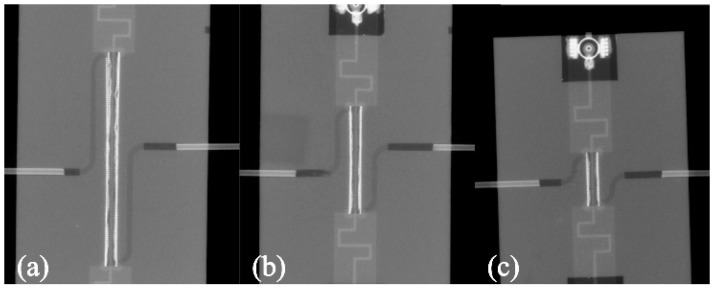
X-ray images of (a) 20 mm, (b) 10 mm, and (c) 5 mm long microreactor.
